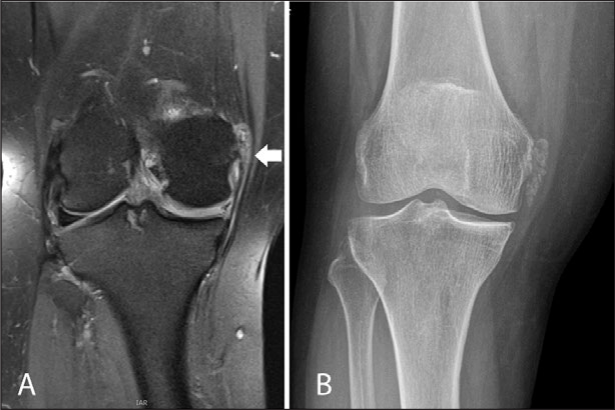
Hydroxyapatite deposition disease (HADD) in the medial collateral ligament in a 66-year-old patient with pain and swelling at the medial aspect of the right knee. Coronal FS T2-WI (A) shows thickening and high signal intensity at the femoral insertion of the medial collateral ligament (arrow). Also note shortening of the body of the medial meniscus, in keeping with a previous medial meniscectomy. Plain films (B) show calcifications at the medial collateral ligament, allowing precise characterization as HADD with surrounding inflammation as origin of the patient’s pain. Calcifications are far better demonstrated on plain radiographs compared to MRI.
